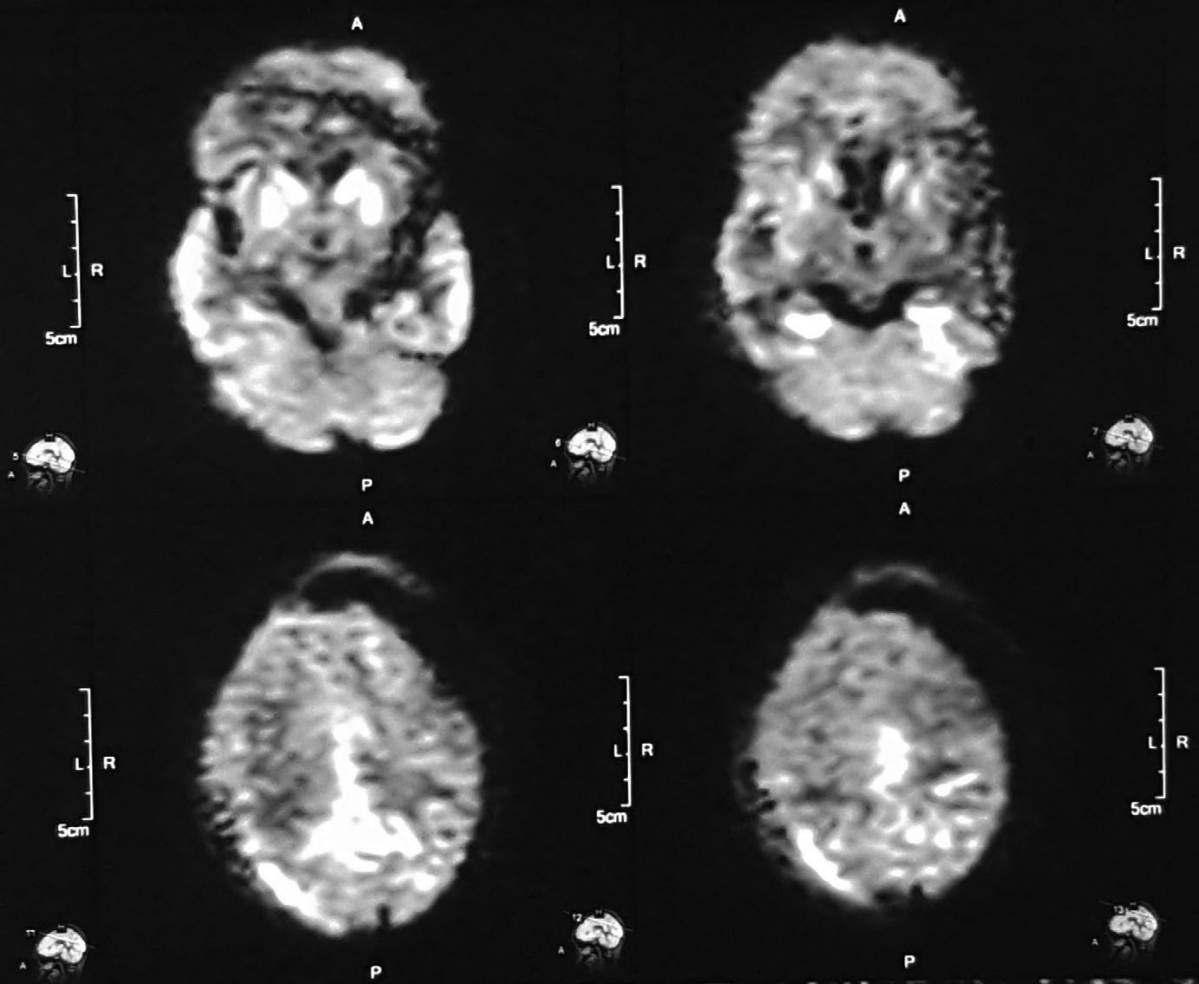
MRI with diffuse‐weighted imaging shows bilateral symmetric basal ganglia hyperintense lesion with cortical ribboning
